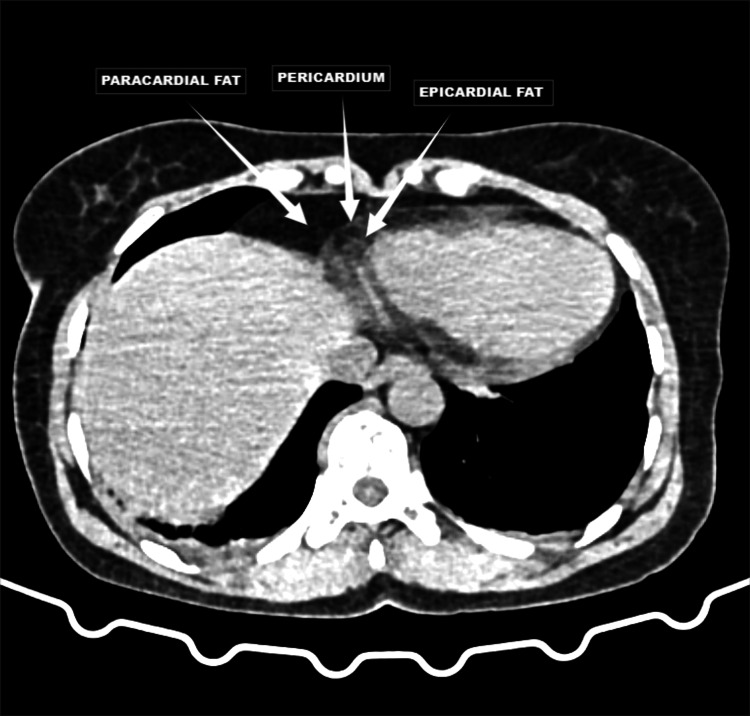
Representative axial non-contrast CT images demonstrating pericardial, paracardial and epicardial fat pads This figure highlights the pericardial fat composed of paracardial fat and epicardial fat separated by pericardium as labeled.
